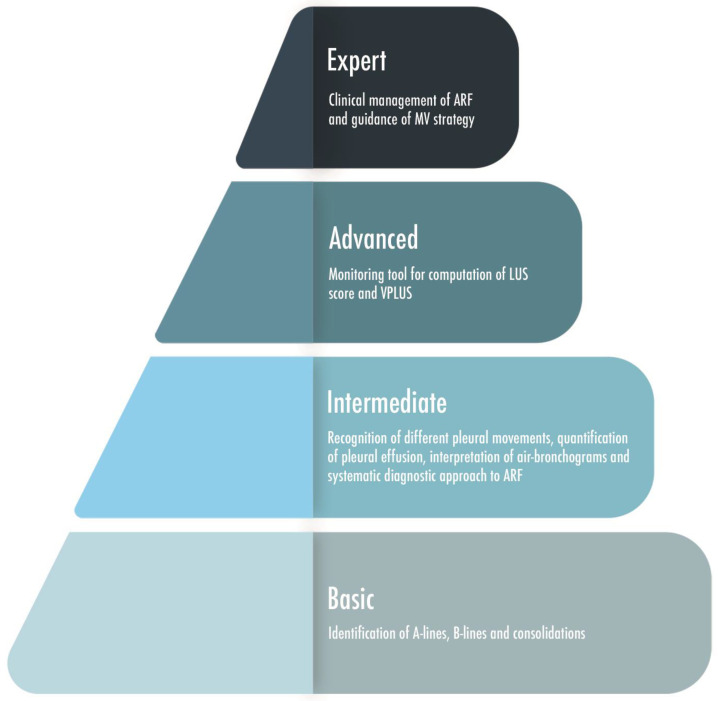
A graphic representation of four level steps of skills-knowledges based on the expertise of the physician performing lung ultrasound. ARF = Acute Respiratory Failure; LUS = Lung Ultrasound; MV = Mechanical Ventilation; VPLUS = ventilator-associated pneumonia lung ultrasound score.
